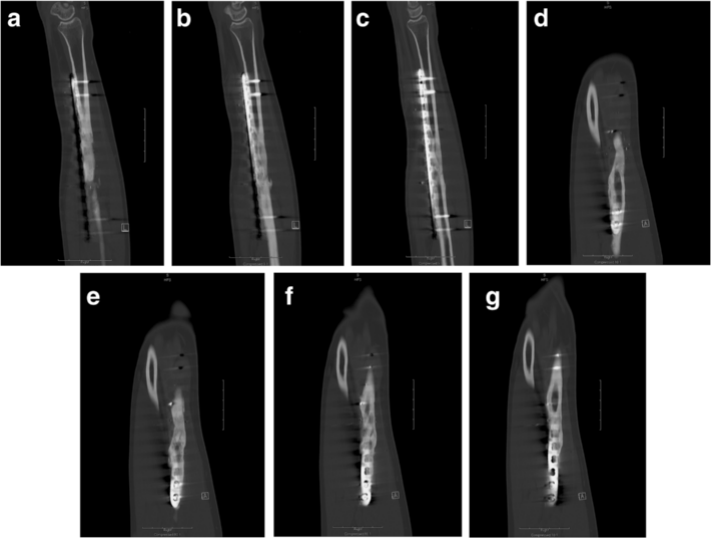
Sagittal (a-c) and coronal CT (d-g) reconstructions of the right forearm one year post-op.
